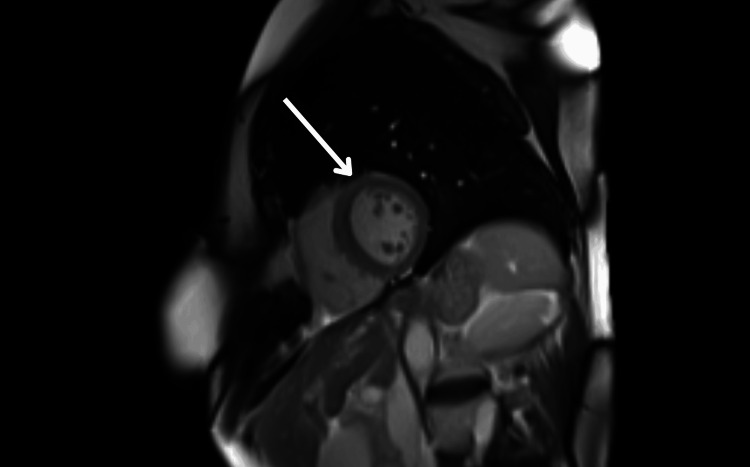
Cardiac MRI demonstrating myocardial inflammation MRI: magnetic resonance imaging
